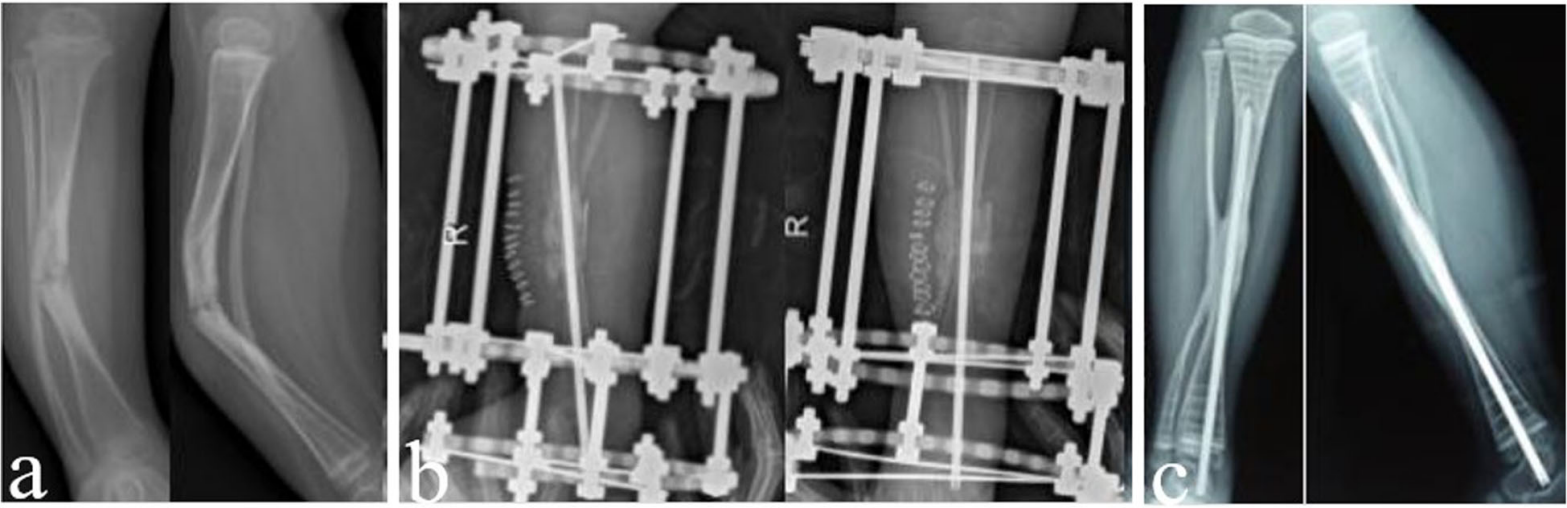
a Preoperative anteroposterior and lateral radiographs. b X-ray radiograph one week post-operation. c Twenty-three months after the operation, X-ray shows that the cross sectional area of the healed segment was enlarged
